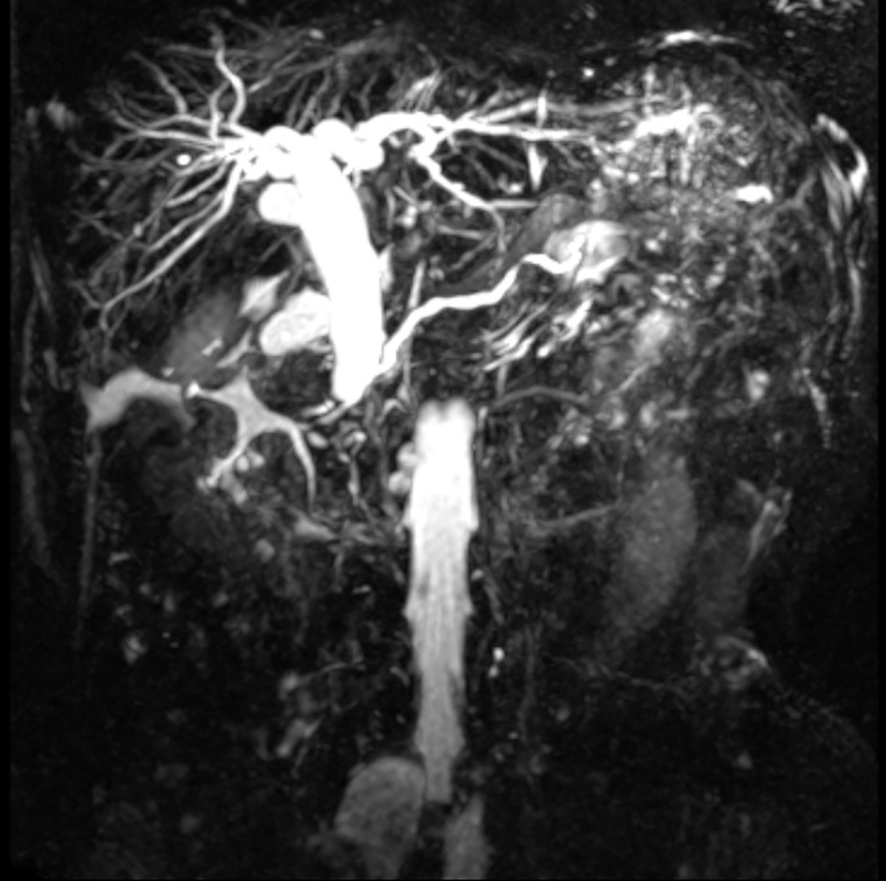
MRI showing dilatation of bile.
